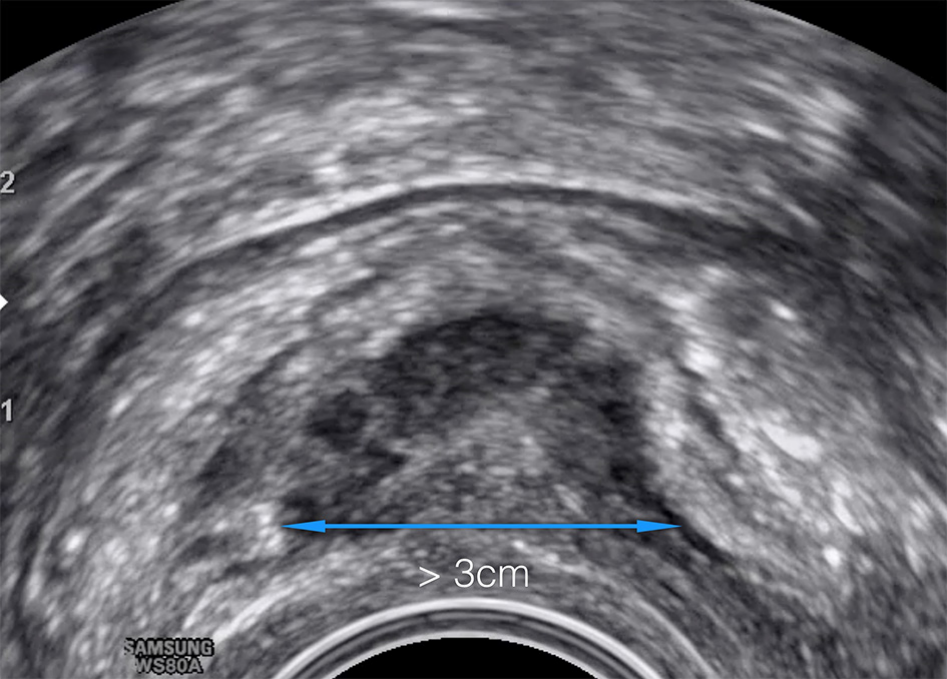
Transvaginal ultrasound of the rectosigmoid with signs of infiltration of the muscular layer. Length >3 cm Enzian C3. Hypodense ultrasound pattern (halfmoon shaped) represents the hyperplasia of the lamina muscularis with inclusion of epithelium, stroma and fibrosis.
